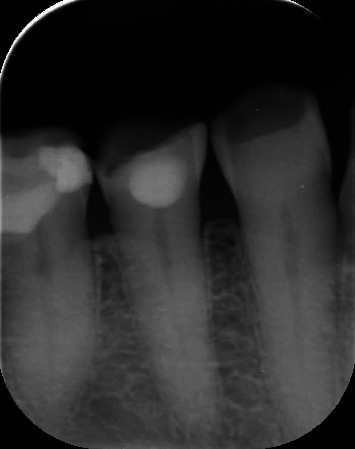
Intraoral periapical radiograph revealing no bone involvement and showing signs of tooth wear.
